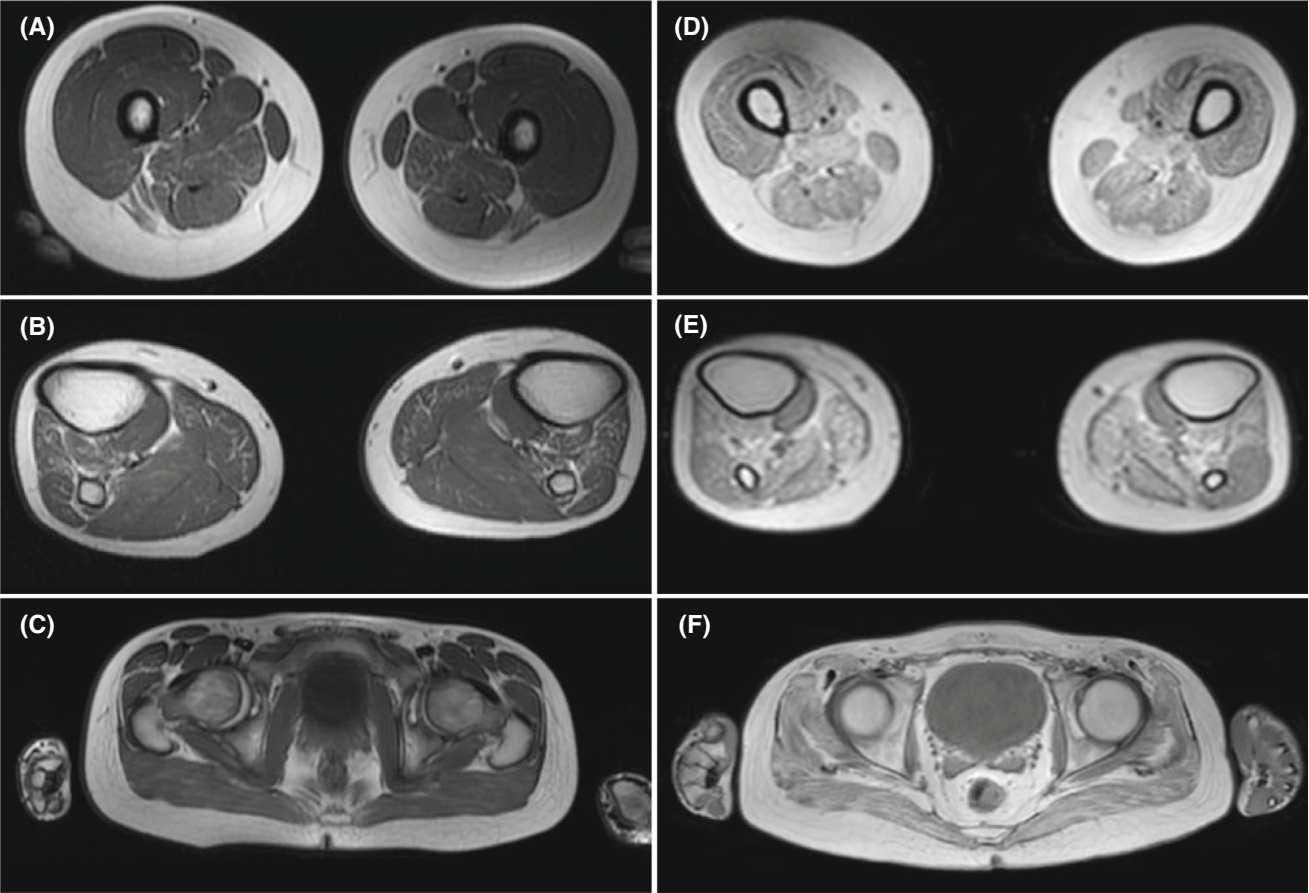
Whole‐body muscle MRI findings of the index case in adolescence. T1‐weighted MRI sequences of lower limb muscles in middle (A–C) and late (D–F) adolescence showed striking progression of fatty infiltration in all muscles. In middle adolescence the fatty infiltration on thighs (A) was observed on adductor magnus; while on calves (B) was more marked on tibialis anterior, extensor digitorum longus, tibialis posterior, and medial gastrocnemius. Muscles of the pelvis (C) were spared. At his 20 sec (D–F) the fatty infiltration and muscle atrophy have significantly progressed on pelvic musculature (psoas, gluteus) and anterior and posterior compartments of both thighs and legs, but also in erector spinae, paravertebral, latissimus dorsi, serratus, abdominal wall muscles, pectoralis, rotator cuff muscles, and biceps. The popliteus muscle (E) was among the very few relatively spared muscles.
